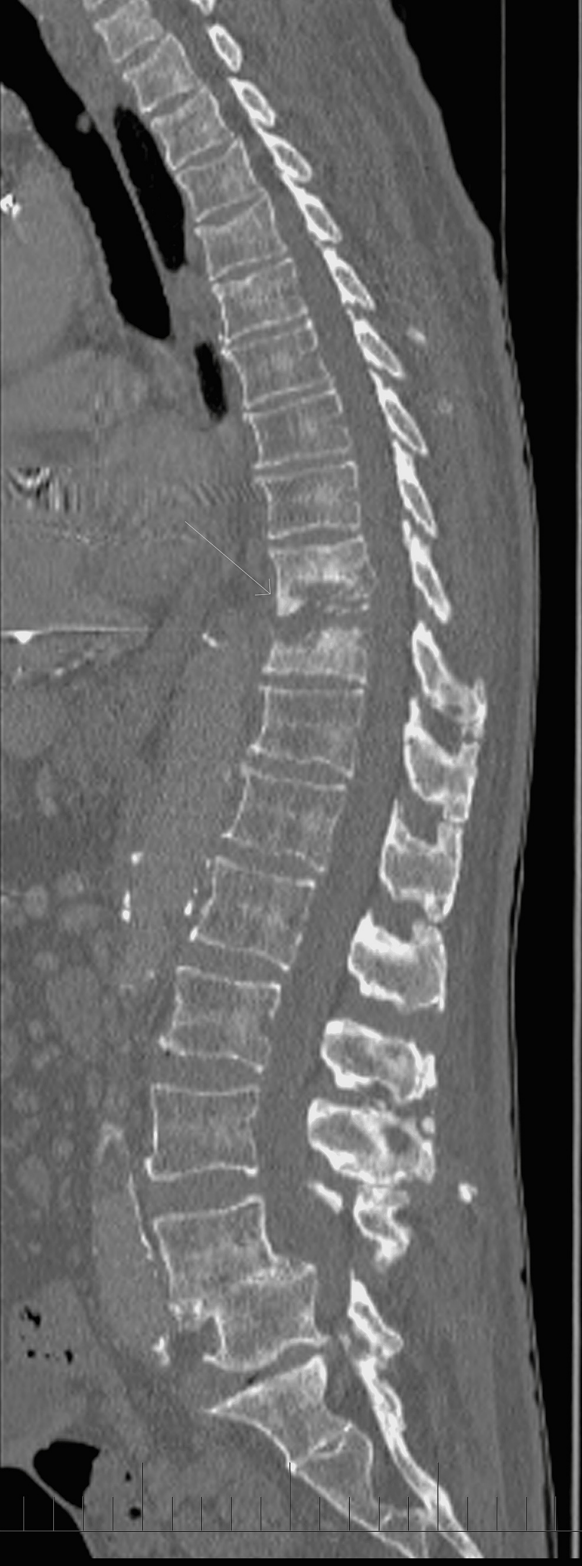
Computed tomography sagittal view of the thoracic spine from the initial visit This demonstrates bony destruction of the T9 and T10 endplates.
